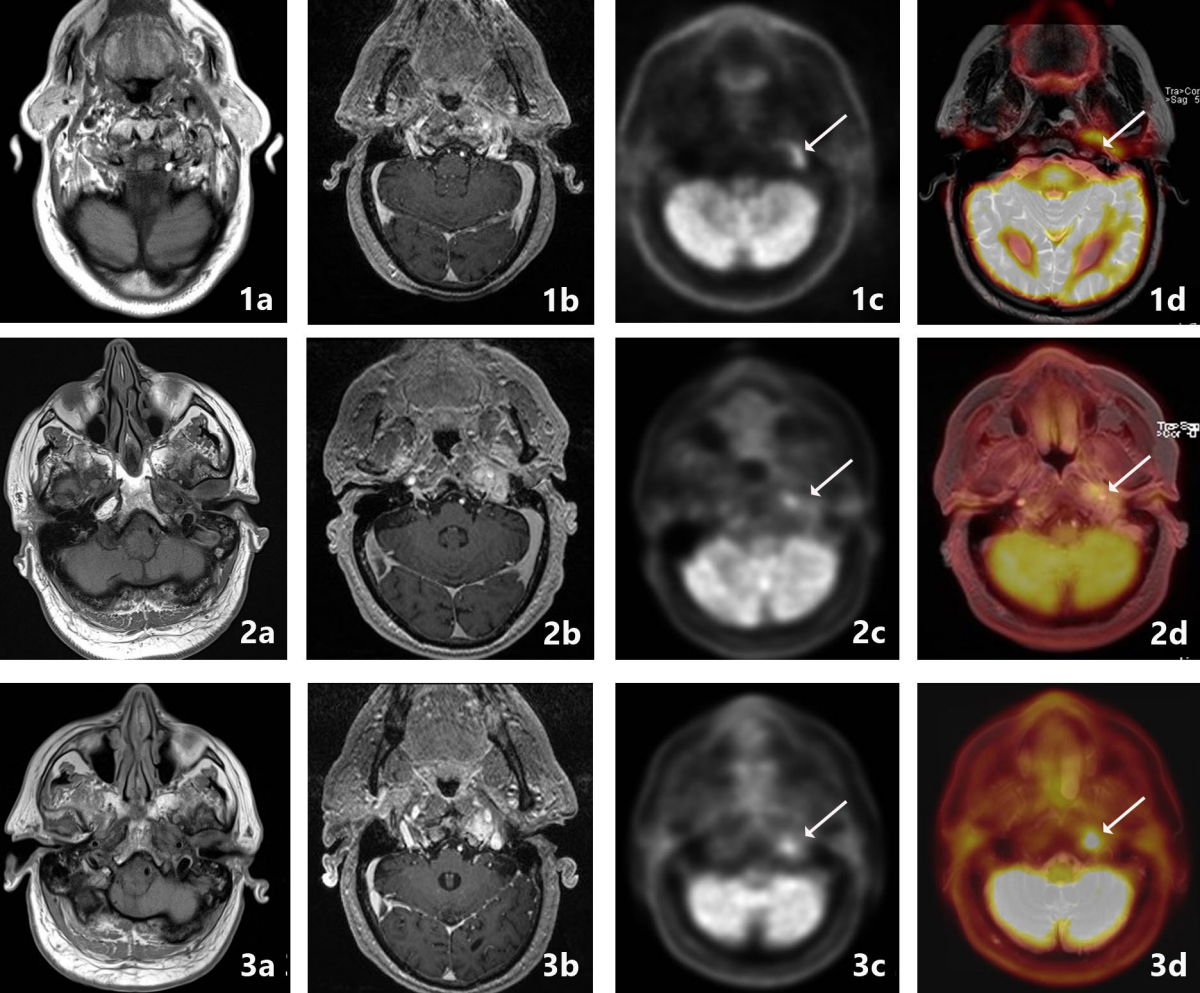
PET-MRI follow-up. A 85-year-old male, with a history of diabetes, presented at a referring hospital 3 months earlier. He was treated with topical and intravenous antibiotics for external otitis. After initial improvement of symptoms, the patient presented with facial nerve palsy and recurrent pain with continuous hearing loss. At CT (not shown) SBO was suspected with obliteration of subtemporal fat planes, induration of the fat at the stylomastoid foramen, osseous destruction of the ventral border of the mesotympanum and malleus. Follow-up was performed with FDG-PET-MRI and additional CT. No increase of bone erosion on CT was noted (not shown). PET-MRI images are shown at 2, 5 and 7 months’ follow-up (respectively, upper, middle and lower panels). From left to right T1-w, contrast-enhanced T1-w, PET and colour fused PET-MRI images are shown. A hypointense signal intensity can be appreciated on the T1-w images (image 2a, 3a, 4a), with loss of fat planes and enhancement after administration of gadolinium (image 2b, 3b, 4b). There is increased uptake of FDG in the temporal bone and subtemporal region, as well as in the retromandibular region (arrows). At follow-up, MRI abnormalities resolved at the meatus and mandibular region with persistent signal abnormalities and enhancement at the medial part of the skull base. The uptake at and below the skull base is decreased at the 5 month follow up, whereas at 7 months there is again an increase in FDG uptake, with new bone marrow edema on T2-w images at the clivus (not shown). Again iv. antibiotics were given; at follow-up at 9 months there was clear improvement in FDG uptake and bone marrow edema. Interestingly enough the increased FDG avidity at the 7-month interval scan (image 3d, 3e) is hardly appreciated on the given images (the scaling of the images was not intentionally adjusted for publication). Showing the importance of quantitative measurement of FDG avidity and comparison with the contralateral side (in accordance with the research of Wong et al.) (Color figure online)
